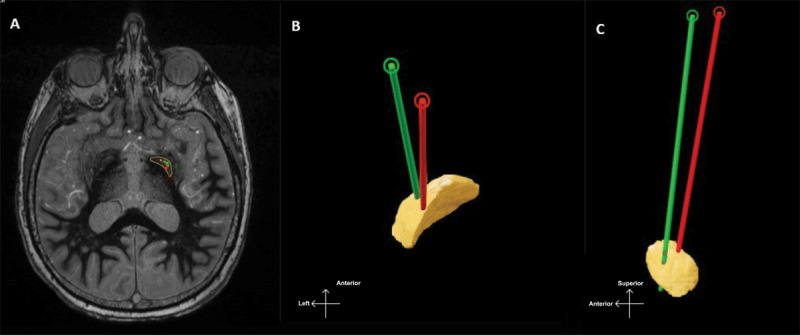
Lead reconstruction of the left GPi DBS lead. The red lead represents the reconstruction performed using the intraoperative CT, when the pneumocephalus and brain shift were present. The green lead represents the reconstruction performed using the post-operative CT (around 1 month later) following resolution of the pneumocephalus and brain shift. A, provides an axial section of a brain MRI collected using the FGATIR sequence. B and C, provide 3D reconstructions of the DBS lead in the left GPi (figure for orientation in the lower left).
